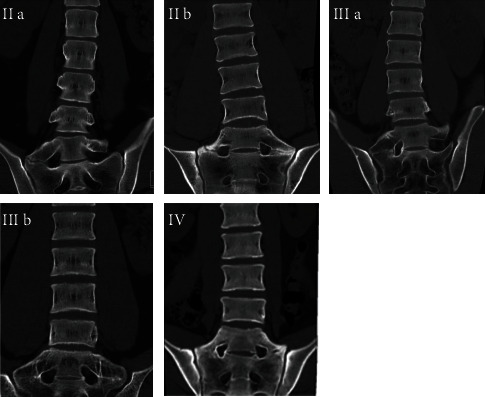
Identification of LSTV. Typical images of Castellvi type IIa, IIb, IIIa, IIIb, and IV are shown on coronal reconstructed two-dimensional computed tomography.
